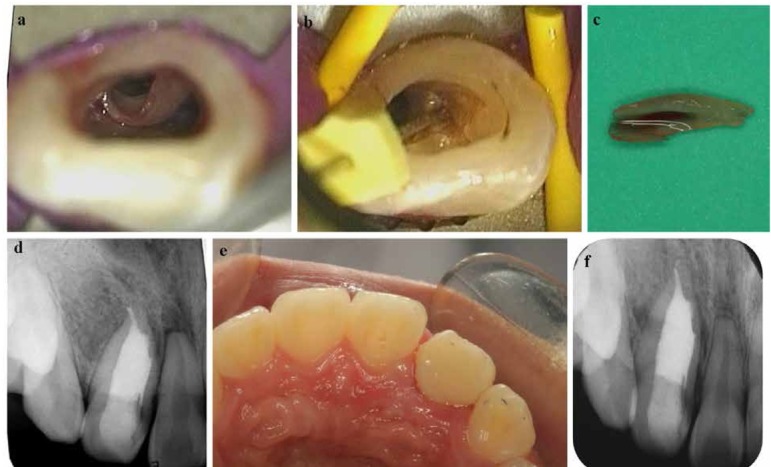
(a) The invaginated structure easily viewed under the DOM after troughing with the ultrasonic tip. (b) H-file used to engage the invaginated structure to remove it from the canal. (c) The removed invaginated structure. (d) IOPA taken after the obturation showing the completely filled canal. (e) Postoperative clinical view of the lingual surface after the restoration. (f) Two- year follow-up IOPA showing the healed lesion.
